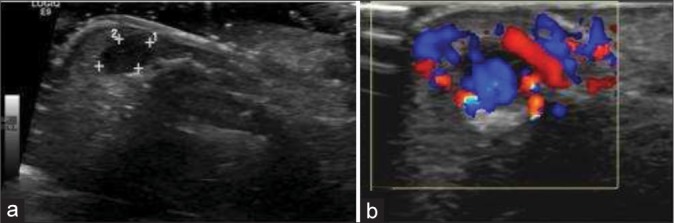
5-year-old boy with soft tissue arteriovenous (AV) malformation of hand who presented with slowly growing swelling of thumb. (a) Gray scale ultrasound image of the tuft of thumb shows a well-defined hypoechoic lesion (measured by calipers), which was misinterpreted as a well-defined cystic lesion. (b) Subsequent Doppler imaging of the lesion demonstrates significant color flow suggestive of AV malformation.
